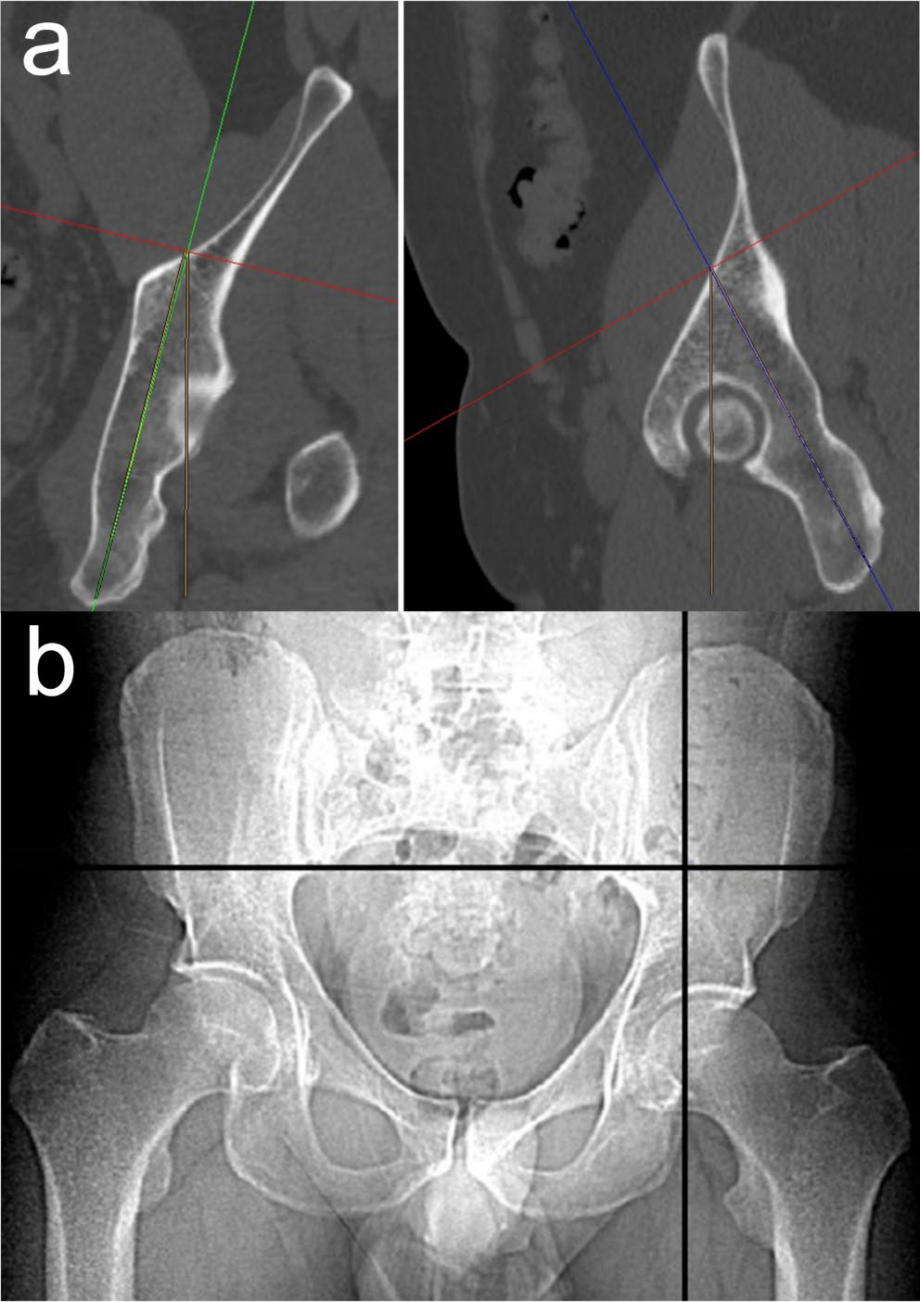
PCS entry point and screw trajectory. a Preoperative planning of the entry point and the screw trajectory of PCS using standard pelvic CT scans and commonly available multiplanar reconstruction tools. The entry point is located at the inner cortex of the iliac bone in the transition zone between the supraacetabular region and the iliac wing. The screw trajectory is typically oriented from cranial–anterior–lateral to caudal–posterior–medial. Coronal reconstruction (left) and sagittal reconstruction (right). b Determination of the entry point using the LiveSync feature in the CT scout image. The CT scout image is performed in a standard supine position and thus corresponds to the intraoperative positioning of the patient
